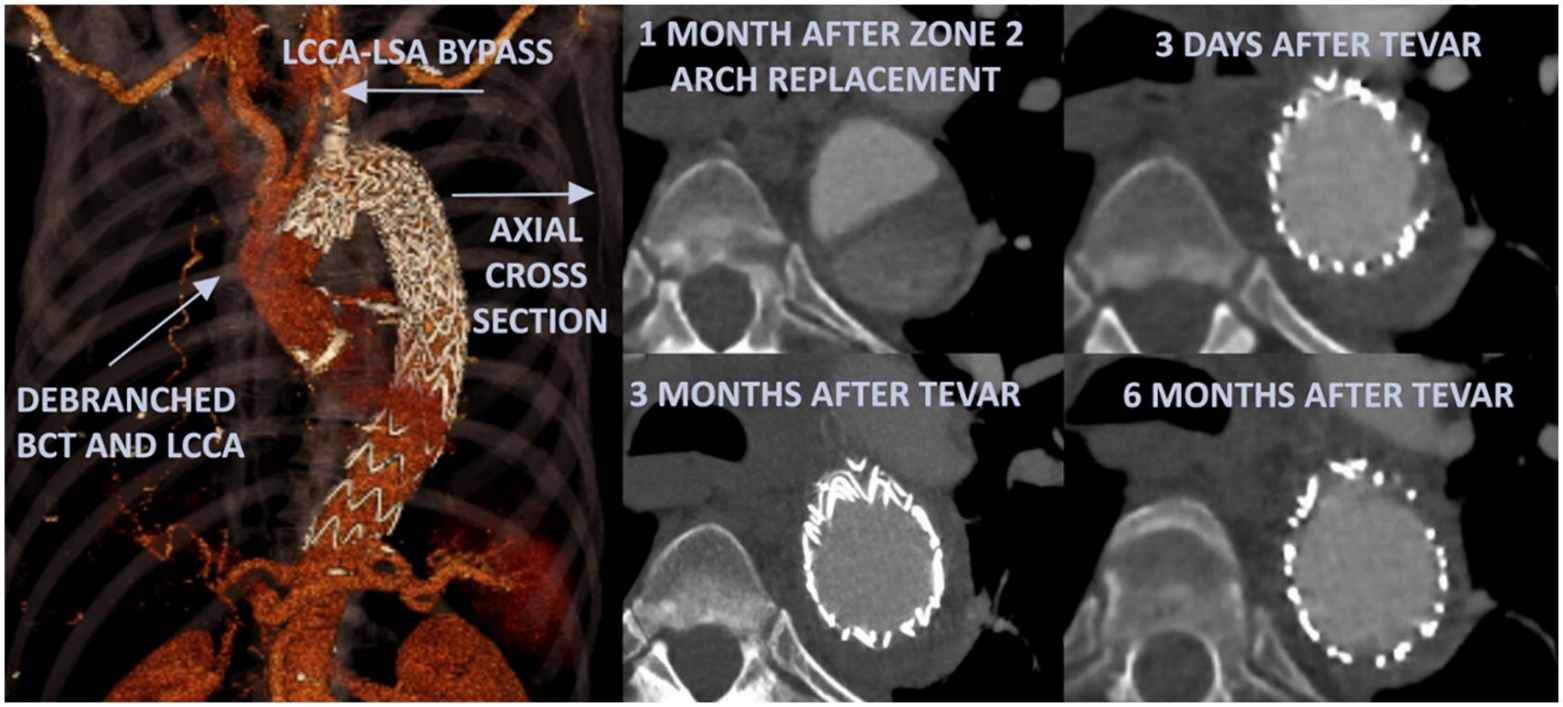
CT reconstruction with cross-sections at different time intervals in a patient after zone 2 arch replacement with debranching of the brachiocephalic trunk and left common carotid artery for acute type A aortic dissection, followed by pre-emptive thoracic endovascular aortic repair.
